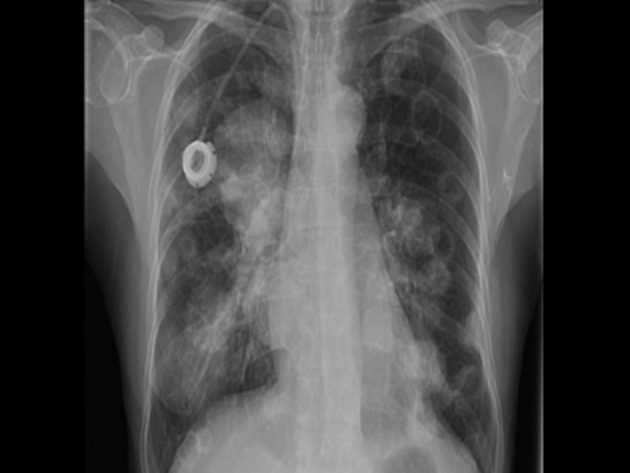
Chest X-ray showing new multiple bilateral cavitary nodules and focal infiltrates in the lateral aspect of the right upper lobe.
